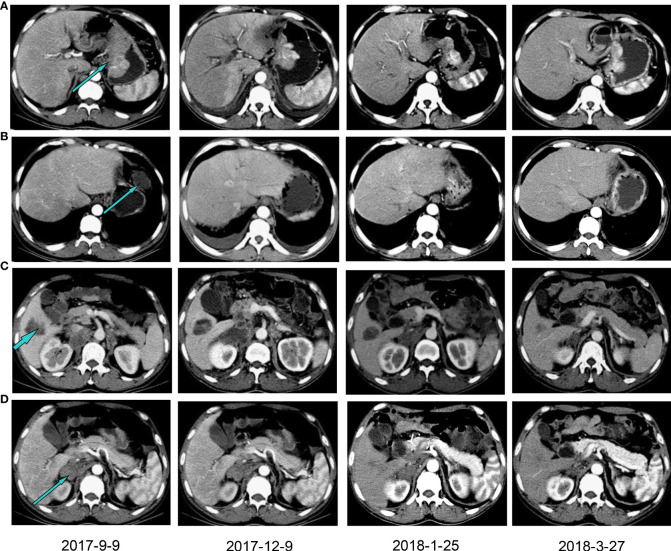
Computed tomography with contrast showing the patient’s lesions before and after PD-1 inhibitor treatment. The lesions in lesser gastric curvature (A), left front of the gastric body (B), right lobe of the liver (C) and right adrenal (D). Patients were treated with a PD-1 inhibitor from December 2017.
